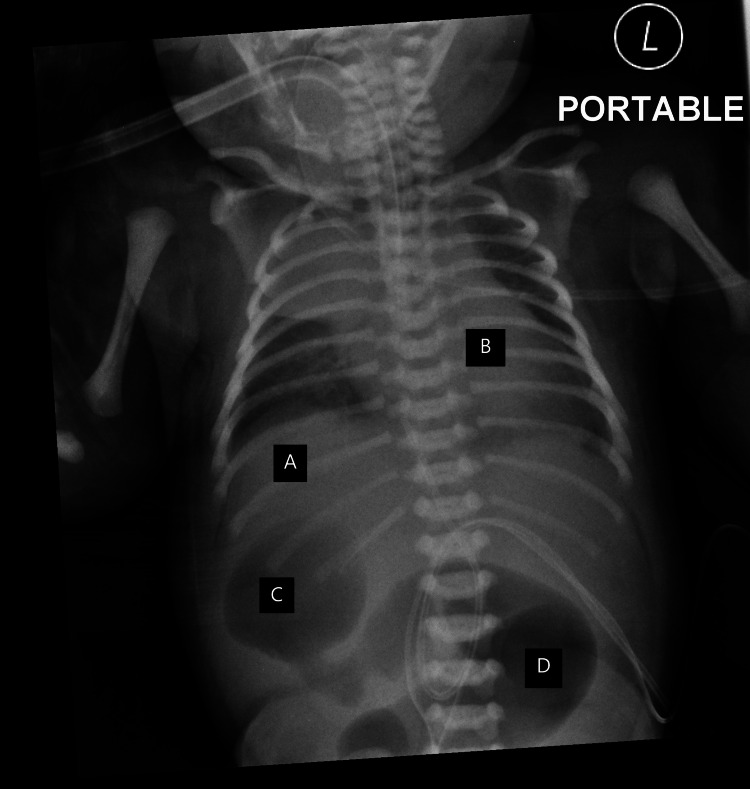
X-ray showing normal cardiac shadow and liver shadow with distended bowel shadows. A: Liver shadow to the right of the abdomen. B: Cardiac shadow to the left of the thorax. C: Gastric bubble to the right of the abdomen. D: Duodenal bubble to the left of the abdomen.
